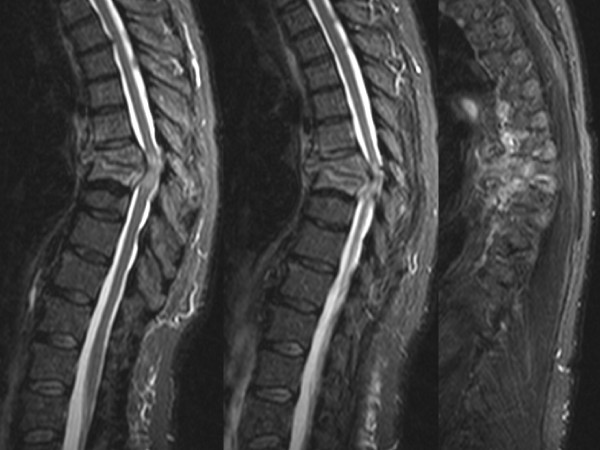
Initial MRI obtained on the first day in the emergency department. STIR sagittal views show the extent of kyphosis at T7/T8 with vertebral body destruction due to pyogenic thoracic spondylodiscitis, spinal canal compression, anterior paravertebral and epidural abscess, and evidence of myelopathy at the T7/T8 level.
